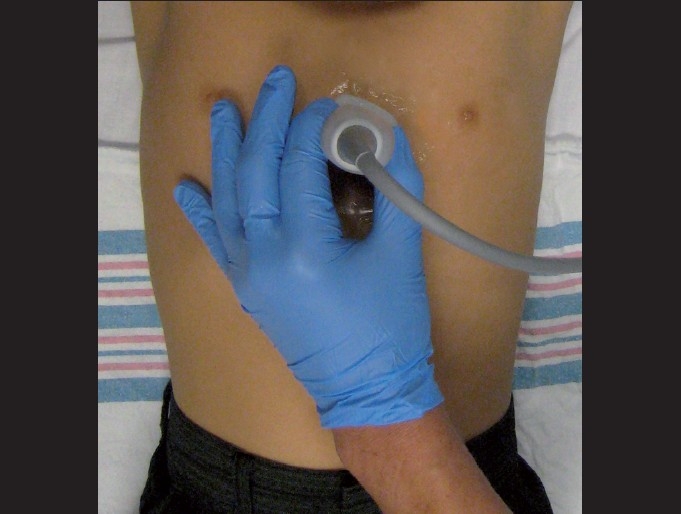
The parasternal long-axis view. The transducer is placed just lateral to the sternum and is oriented with the probe marker toward the patient’s left hip, or in the 4:00 direction, perpendicular to the patient
